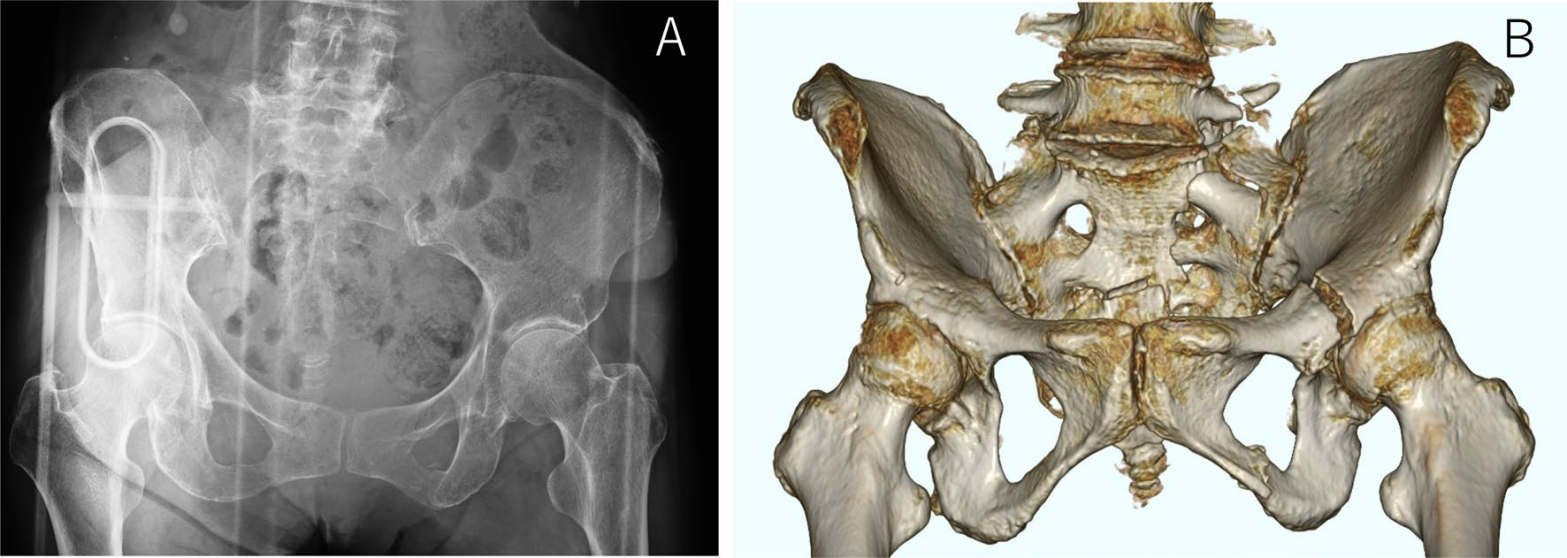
Preoperative X-rays and computed tomography (CT). Pelvic anteroposterior (AP) view (a). Preoperative three-dimensional CT reconstruction of AP view (b)
